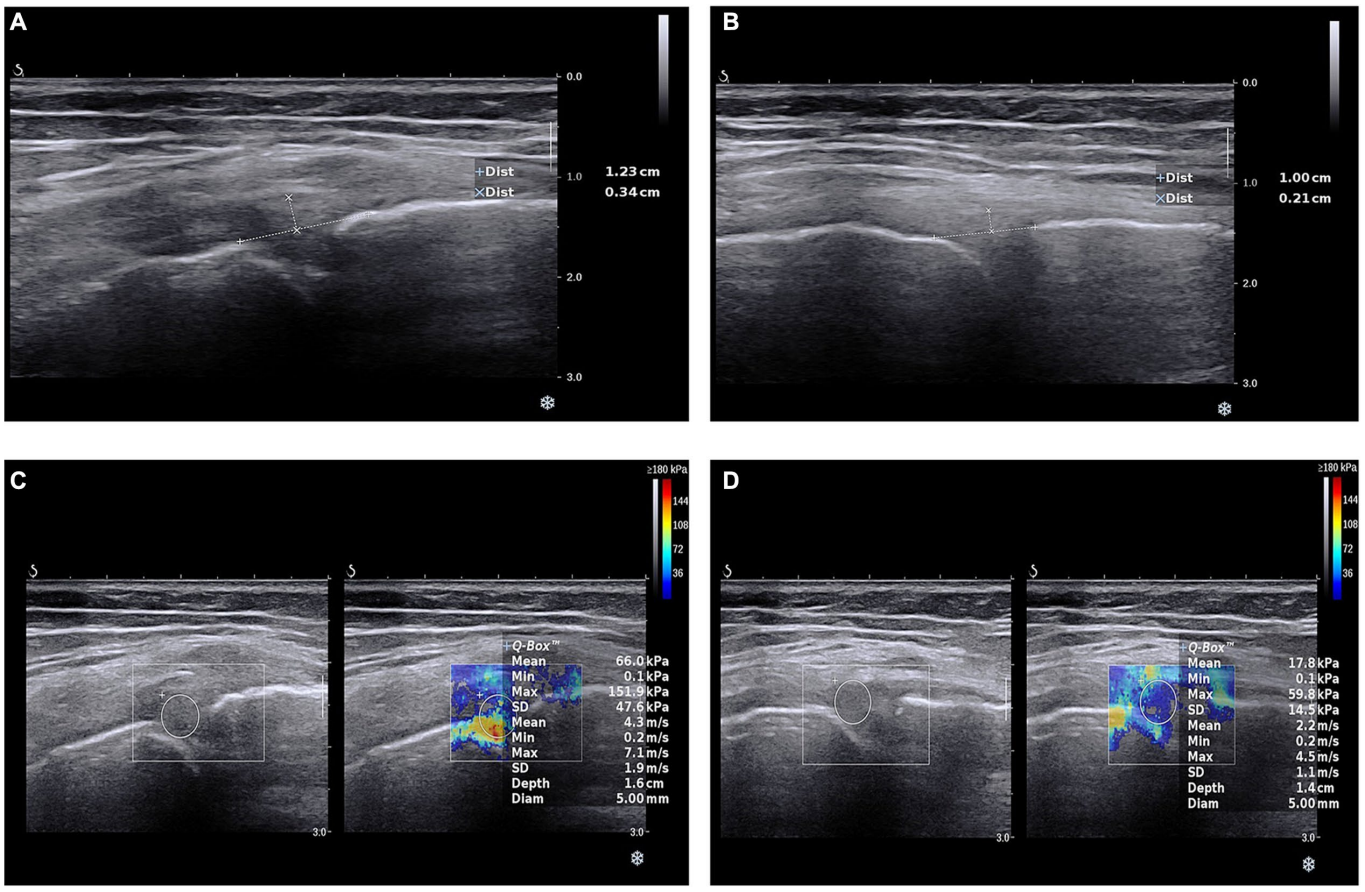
Ultrasonic shear wave elastography images. (A) Medial meniscal projection distance in osteoarthritis patients. (B) Medial meniscal projection distance in health people. (C) Medial meniscus elasticity in osteoarthritis patients. (D) Medial meniscus elasticity in health people.
